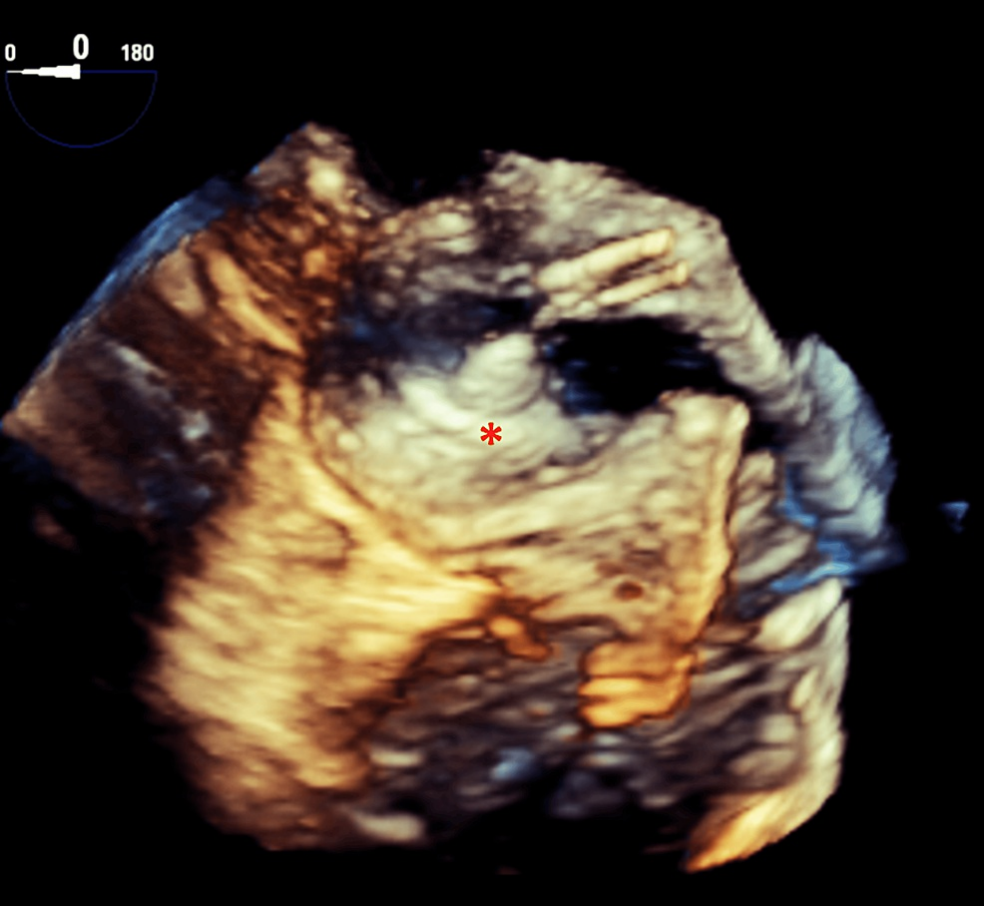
Transesophageal echocardiogram showing 27 mm Watchman FLX positioned in the LAA (surgeons view). * = 27 mm Watchman FLX occluding the left atrial appendage
